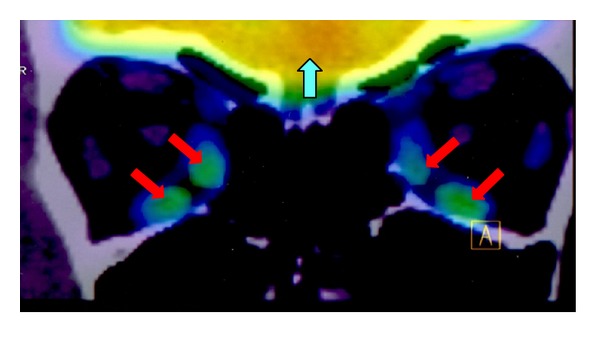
PET/CT coronal view of extraocular muscles. Warm to cold color scale (red, orange, yellow, green, blue, and purple) shows a high to low FDG uptake (accumulative radioactivity). The image shows high FDG uptake from brain (blue arrow) and an increased FDG uptake in inferior and medial recti muscles (red arrows).
